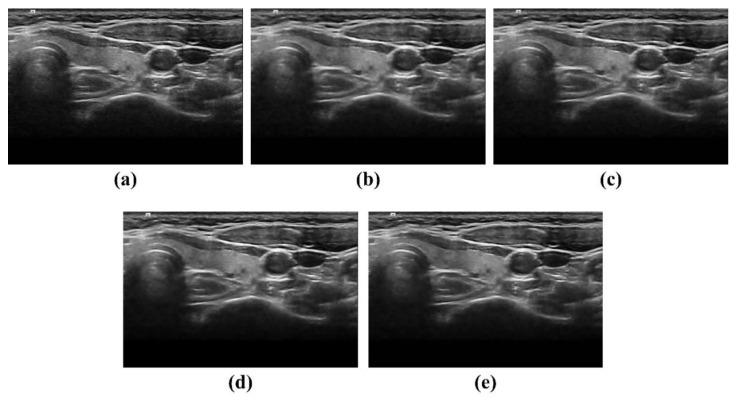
Acquired human thyroid ultrasound images captured using (a) noisy, (b) median filter, (c) Wiener filter, (d) PGPD, and (e) proposed non-local means noise-reduction algorithm.
